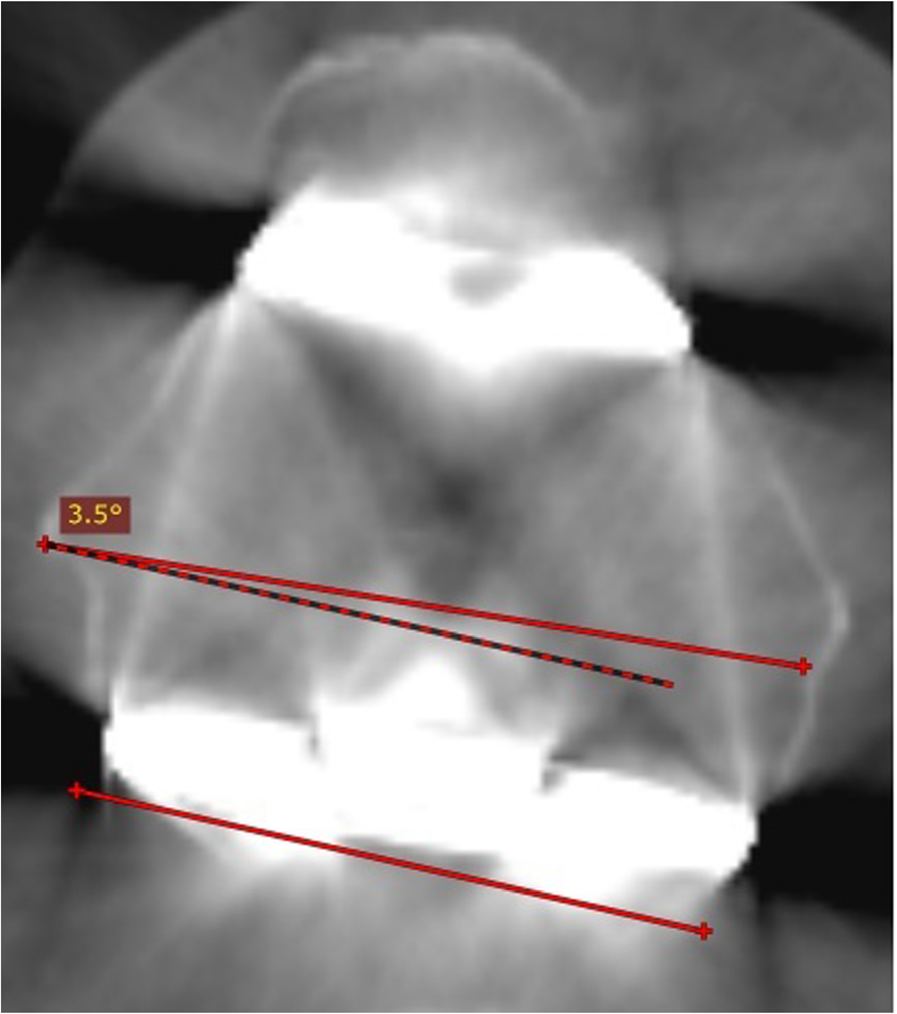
Axial computed tomography slice of a total knee arthroplasty in a male patient, showing neutral femoral component rotation in relation to the surgical transepicondylar axis (sTEA). The sTEA is plotted as a line drawn from the sulcus of the medial epicondyle to the prominence of the lateral epicondyle. The posterior condylar line of the femoral component is plotted as a line drawn from connecting the posterior margins of the medial and lateral posterior component condylar surfaces. The angle between these two lines was then measured and is the posterior condylar angle
